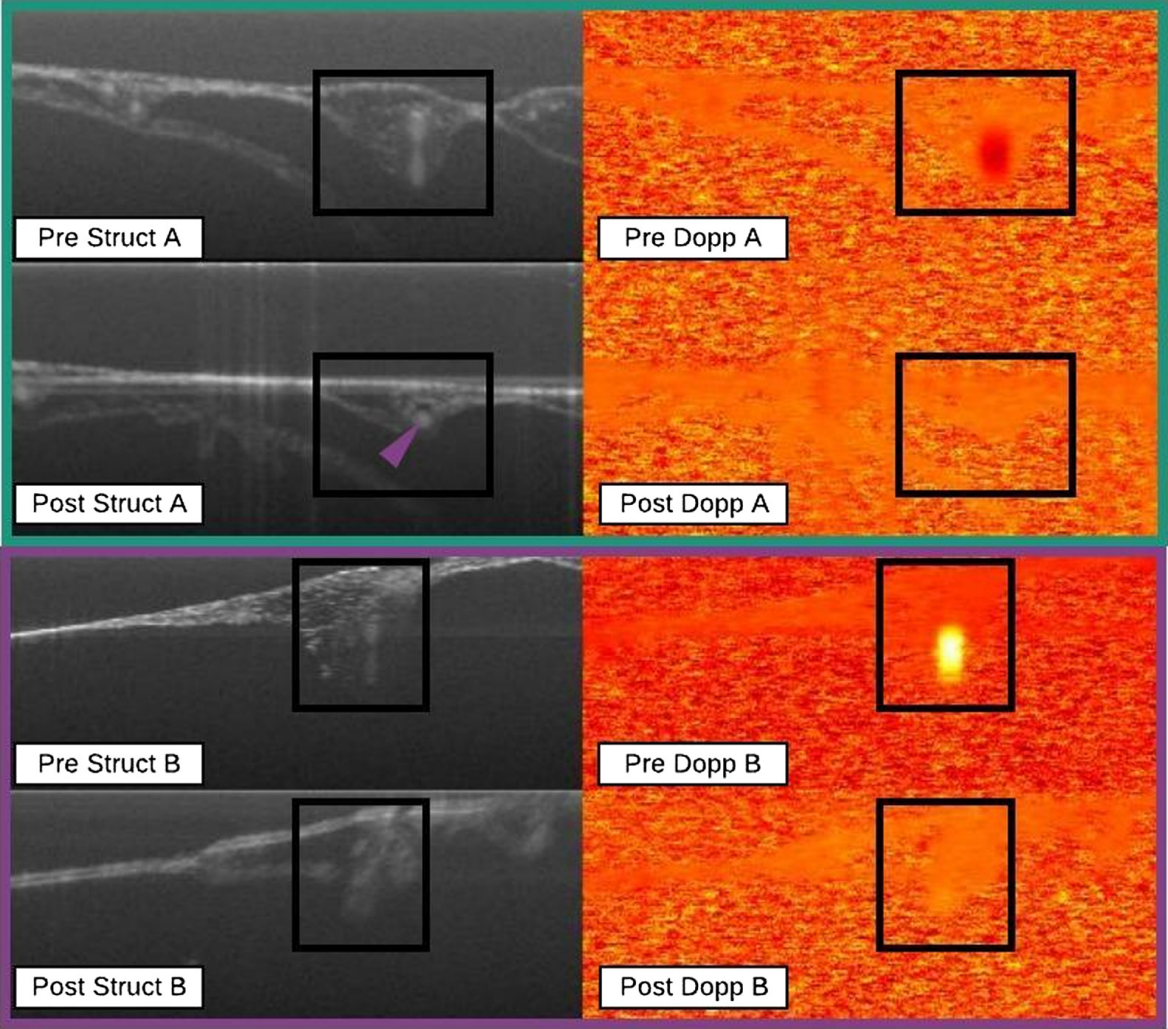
Seal data points A and B. Evidence of embolism (green rectangle) and significant morphological change (purple rectangle). Black boxes represent ROIs and the purple triangle indicates uniform structural signal within vessel lumen suggesting embolism. In all data points note the disappearance of the lemniscate morphology from pre- to poststructural images as well as the disappearance of the Doppler signal in the post-ROI.
